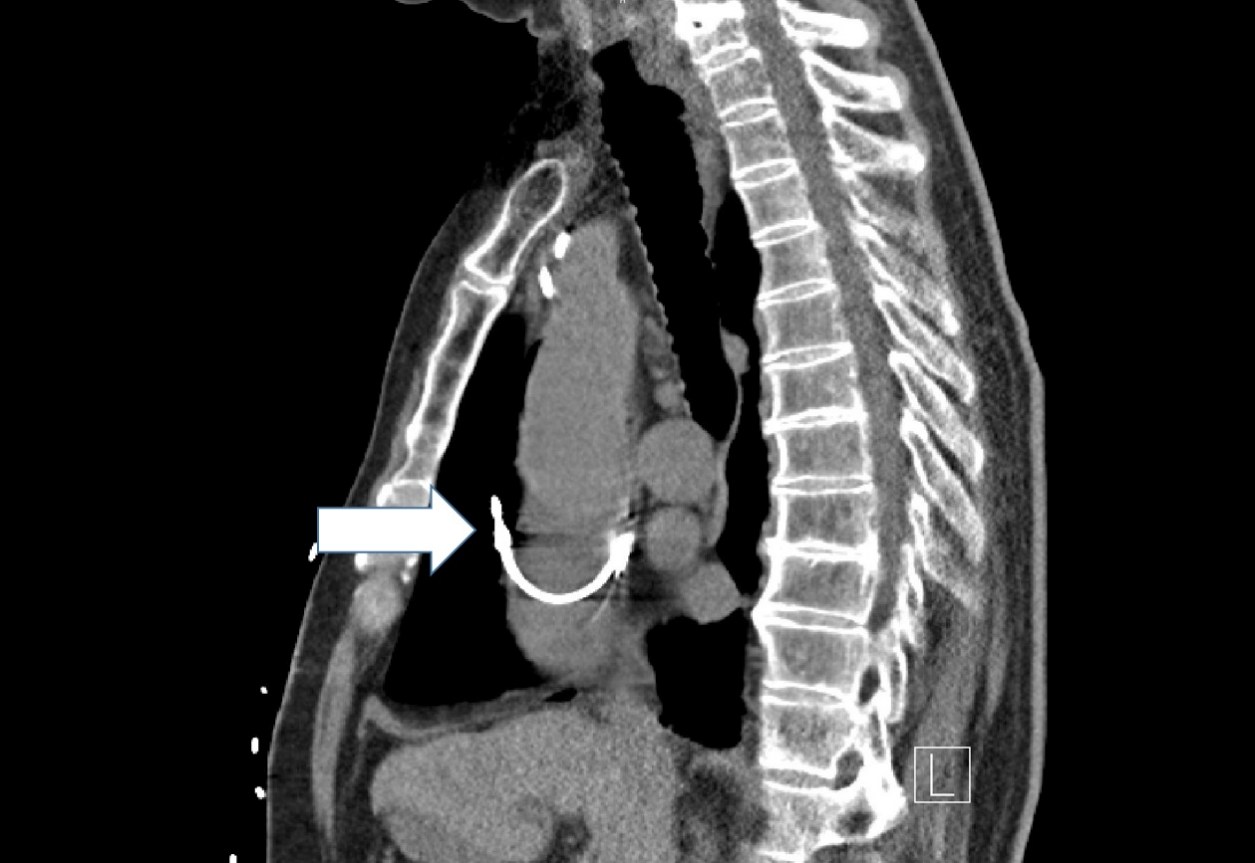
High-resolution computed tomography scan of the chest sagittal cut with the white arrow pointing at the tip of the atrial lead migrating into the right lung
